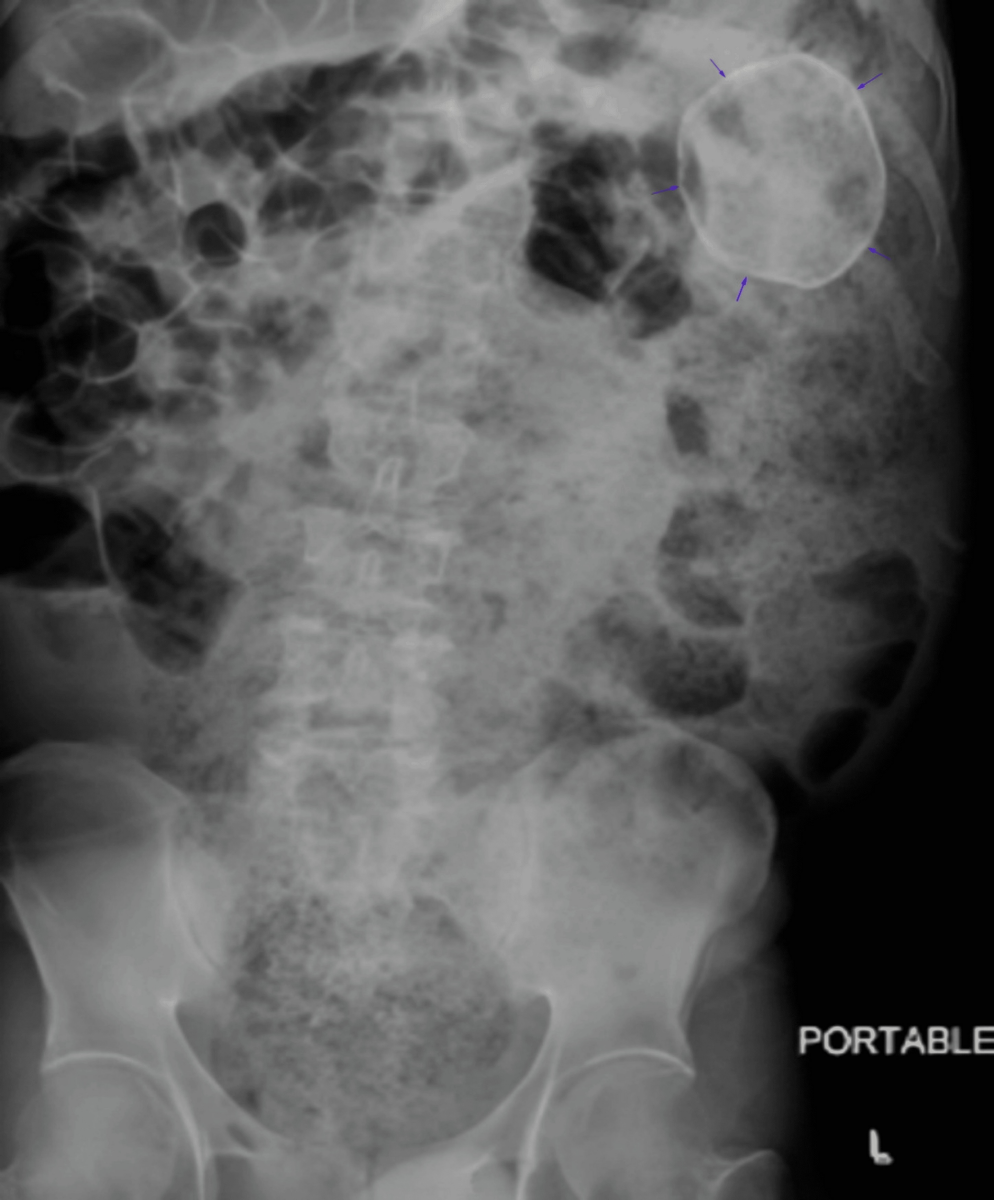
Plain film abdominal X-ray demonstrating movement of the fecalith (arrows) into the proximal descending colon.
